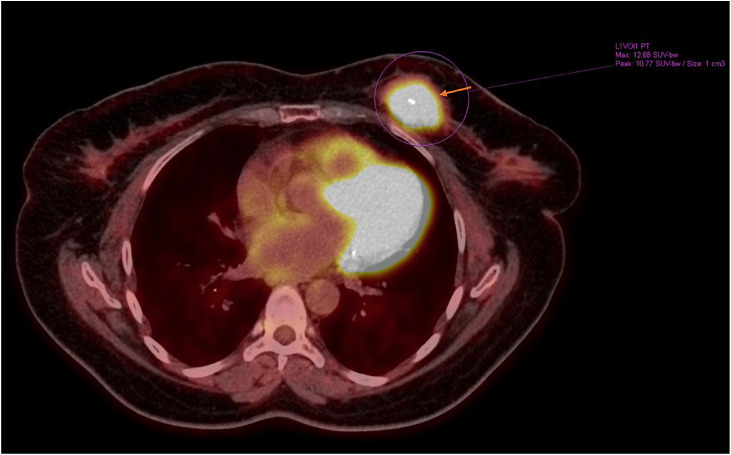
Whole-body 18F-FDG PET scan demonstrated a hypermetabolic, irregular soft tissue mass in the upper outer quadrant of the left breast, with a biopsy marker in place (arrow).
